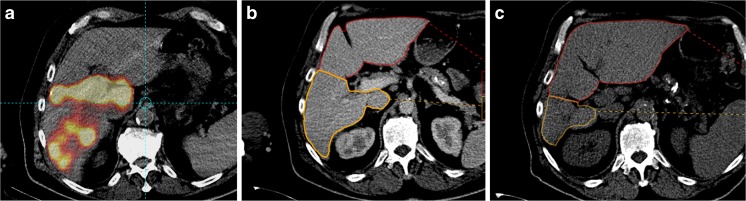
Example of hypertrophy related to a high TD for a large tumor for a patient with a very low HILD. This 66-year-old man with a Child A6 cirrhosis with a large unifocal HCC recurrence after chemoembolization was treated with 1.5 GBq of ^90^Y–loaded glass microspheres injected in the RHA. The ILD was 130 Gy, HILD was 21 Gy only while TD was 361 Gy for a tumoral volume of 151 CC. The MAA scintigraphy confirmed a perfusion of segment 1 (a). The initial hepatic reserve was 39% (b). At 6.5 months after RE, we observed a hypertrophy of 82% of the left lobe and a 52% atrophy of the right treated lobe. The hepatic reserve was then 71% (C)
